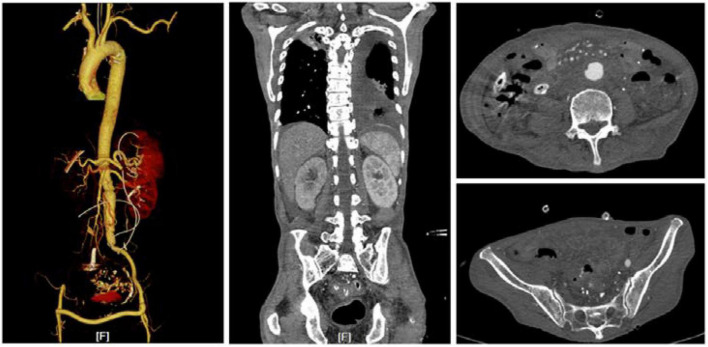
CTA result at 1 month after the operation. Abscesses in the abdomen were cured. The bypass vessels and the lumen were unobstructed.
